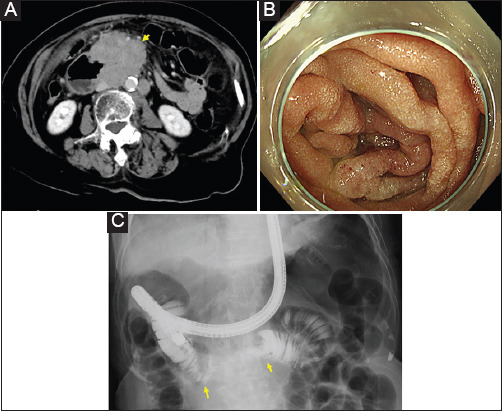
Enhanced abdominal CT revealed a severely dilated stomach and significant dilatation of the duodenum up to the level of the third portion, which was compressed by a large, low-density mass, suggestive of malignant lymphoma (arrow) (A). Upper gastrointestinal endoscopy showed narrowing of the third portion of the duodenum with edematous mucosa covered with multiple white spots, where the endoscope was able to pass through with mild resistance (B). Fluoroscopy showed severe stricture at the third portion of the duodenum (arrows) (C).
